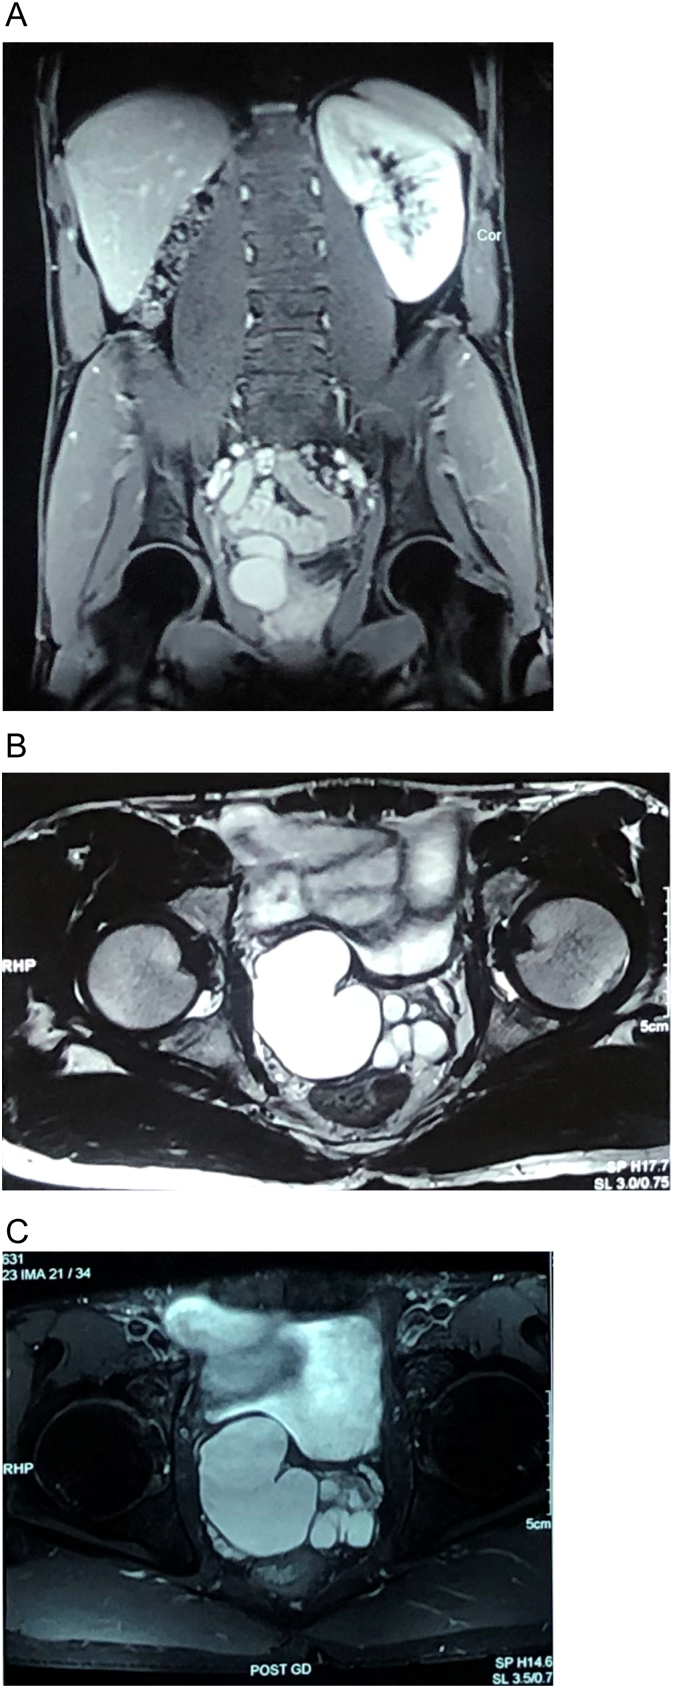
A: MRI abdomen and pelvis, T1, coronal. B: MRI abdomen and pelvis, T1, transverse. C: MRI abdomen and pelvis, oblique.
